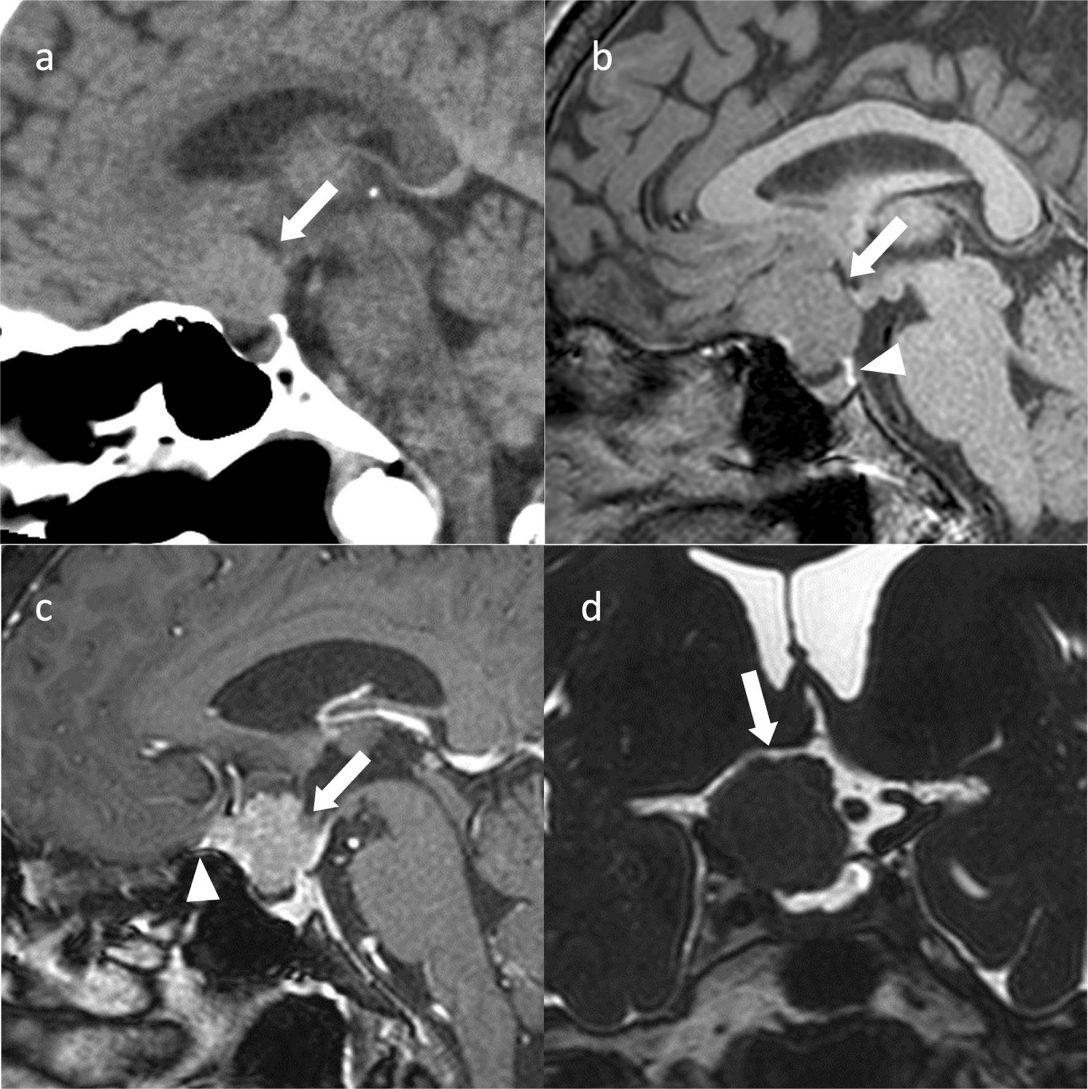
Tuberculum sellae meningioma (meningothelial meningioma). An approximately 60-year-old female with decreased right visual acuity. a Sagittal non-contrast computed tomography showing a mildly hyperdensity mass compared to white matter in the suprasellar region (arrow). No obvious thickening of the bone adjacent to the mass is observed. b On sagittal T1WI, the mass shows isointensity compared to gray matter (arrow). Normal posterior pituitary gland hyperintensities are observed (arrowhead). c Sagittal contrast T1WI shows uniform and strong contrast enhancement (arrow). The dural tail sign is observed anterior to the mass (arrowhead). d Coronal heavy T2WI shows compression of the right optic nerve by the mass (arrow). Craniotomy was performed, and the tumor was confirmed as a meningothelial meningioma
